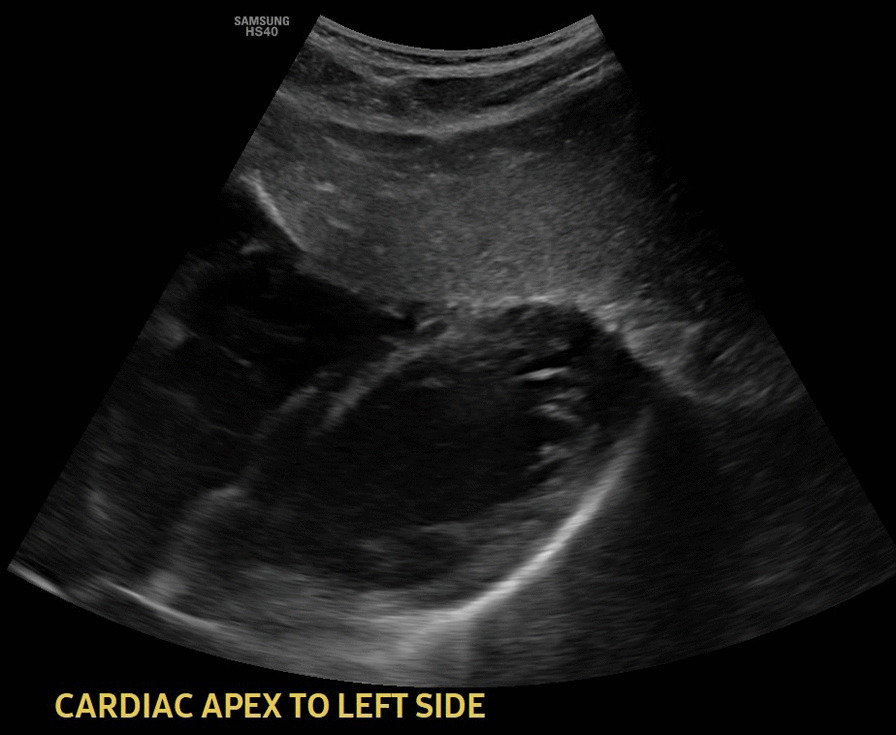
Ultrasound image of cardiac apex positioned toward the left
